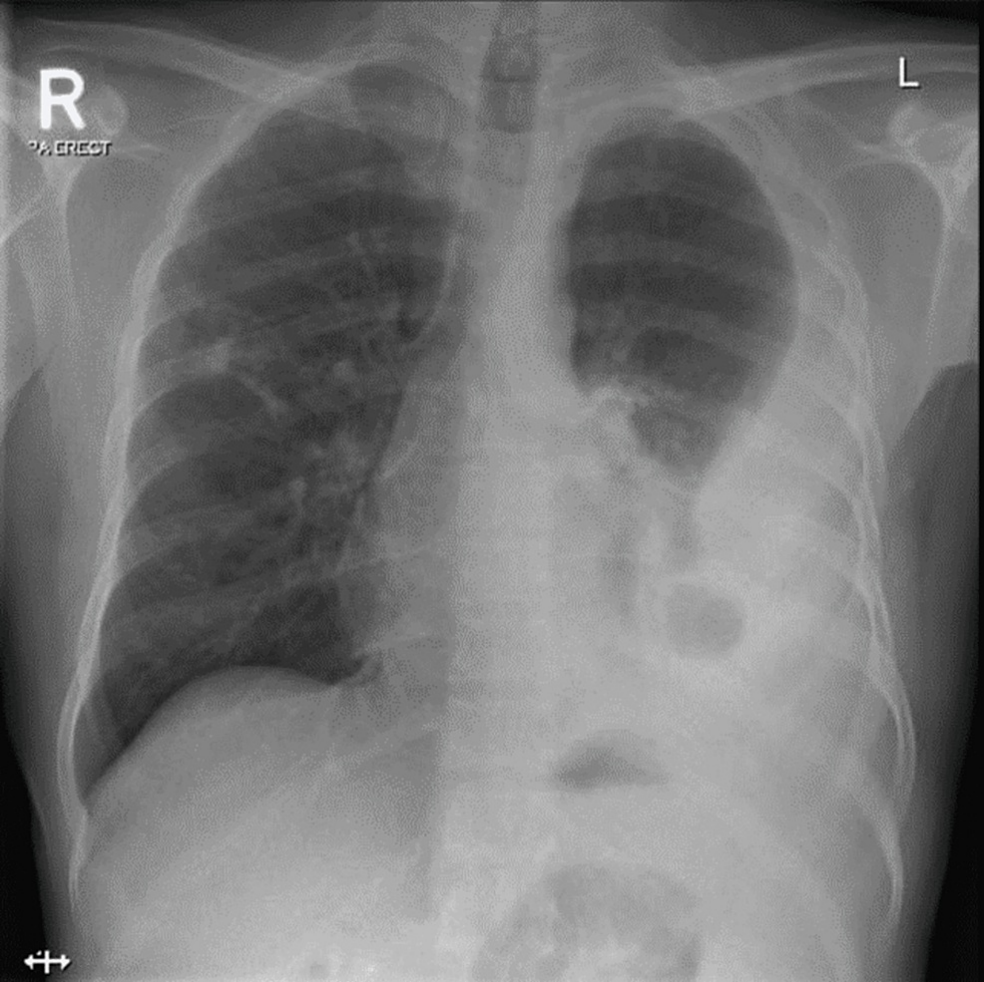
Chest X-ray showing large left-sided pleural effusion with collapse-consolidation of the left lung lower lobe. A patchy nodular opacity is seen in the right lung mid-zone
